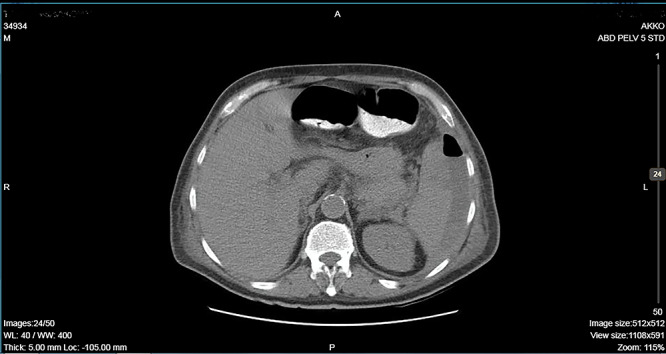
CT showing an important decrease of the abscess due to its rupture toward the peritoneal cavity and presence of air in the abdominal cavity.
